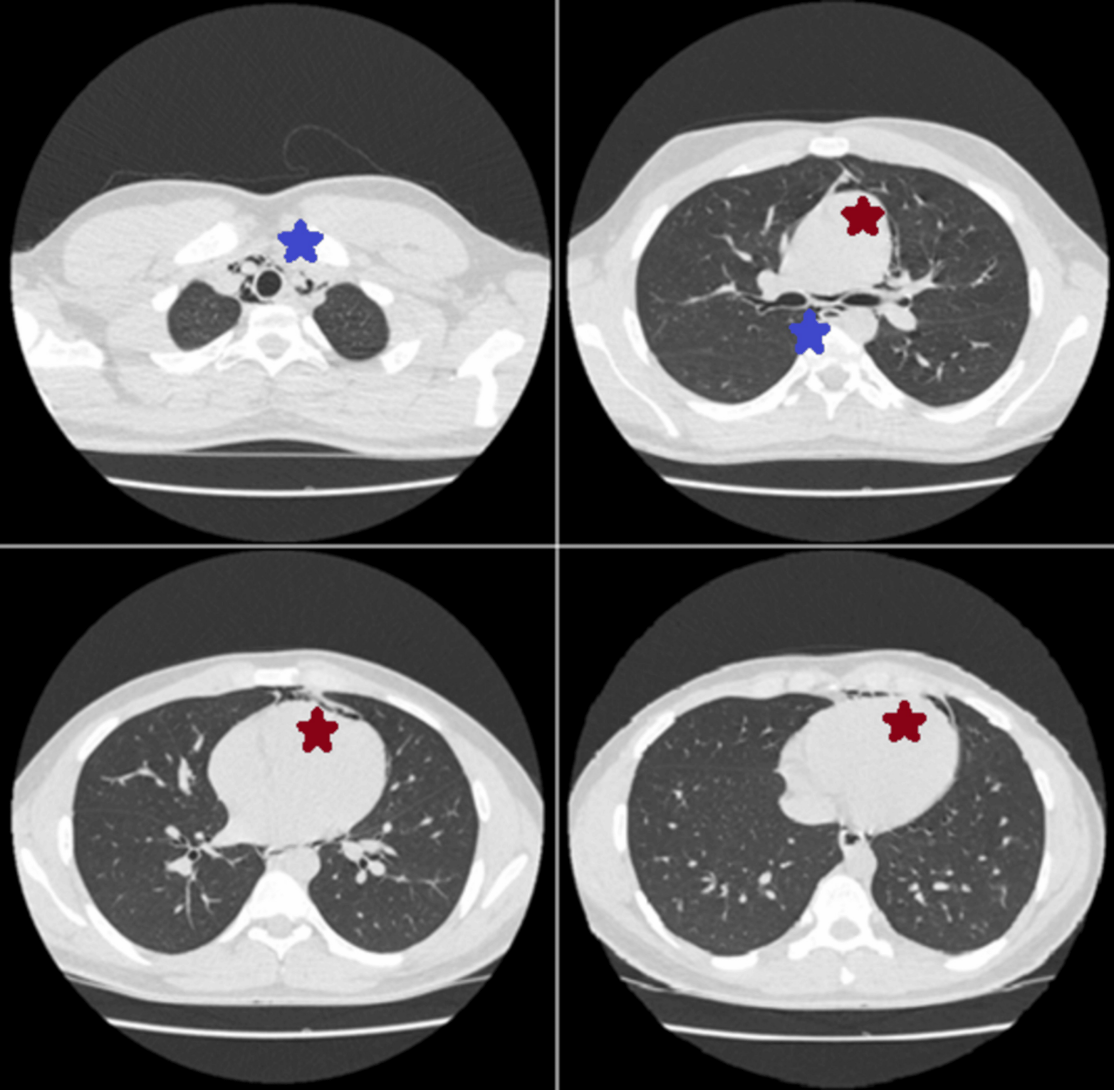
Chest CT showing free air within the mediastinum (blue asterisks) and the pericardial cavity (red asterisks).
